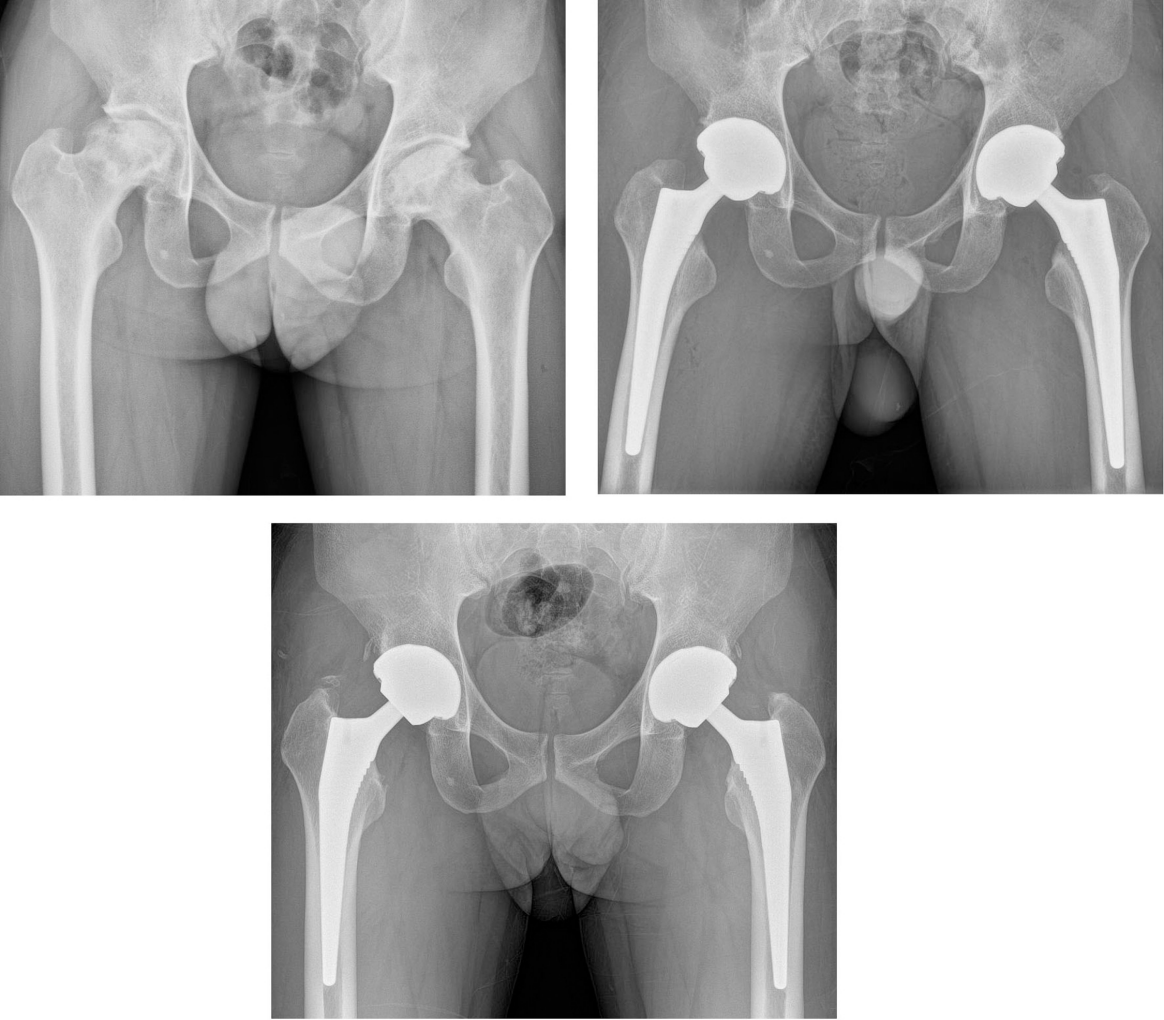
(A) Preoperative hip radiograph of a 43-old man with osteonecrosis of the femoral head involving both hips. (B) Simultaneous bilateral total hip arthroplasty was performed with cementless acetabular components and tapered wedge femoral stems. Closed suction drainage was not used for this case. Calculated blood loss was 1254 mL, and allogenic blood transfusion was not performed. (C) A 4-year postoperative radiograph showed stable implant fixations. The patient was able to participate in daily activities without pain and was satisfied with the surgical outcome.
